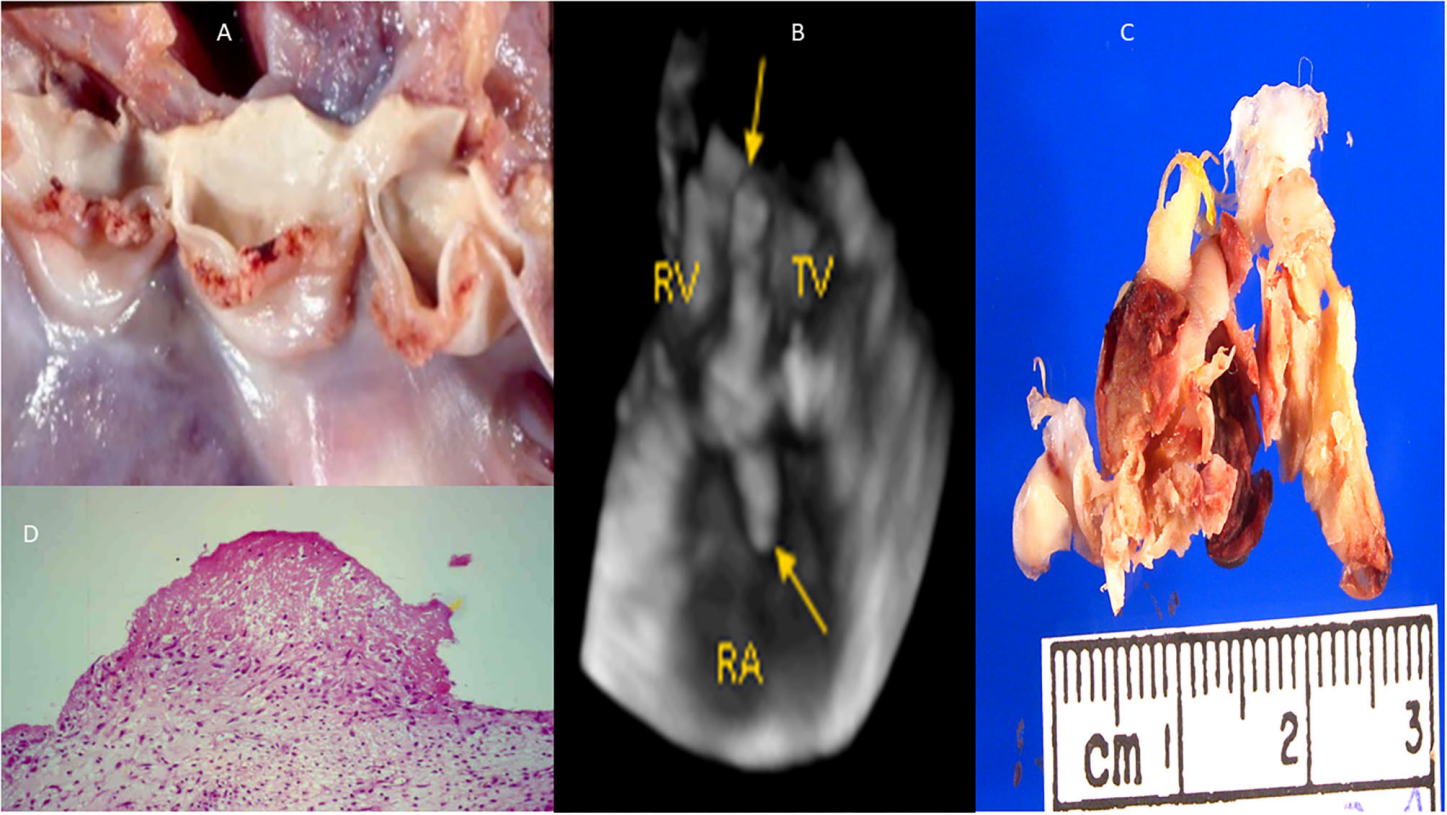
Infective endocarditis (aortic valve and tricuspid valve). (A) Macroscopy: small vegetations all over the aortic valve. (B) 3D transthoracic echocardiography depicting vegetations over the tricuspid valve (arrows). (C) Macroscopy: vegetations all over the tricuspid valve. (D) Microcospy: small fibrine thrombi in organization. RV, right ventricle; RA, right atrium; TV, tricuspid valve.
